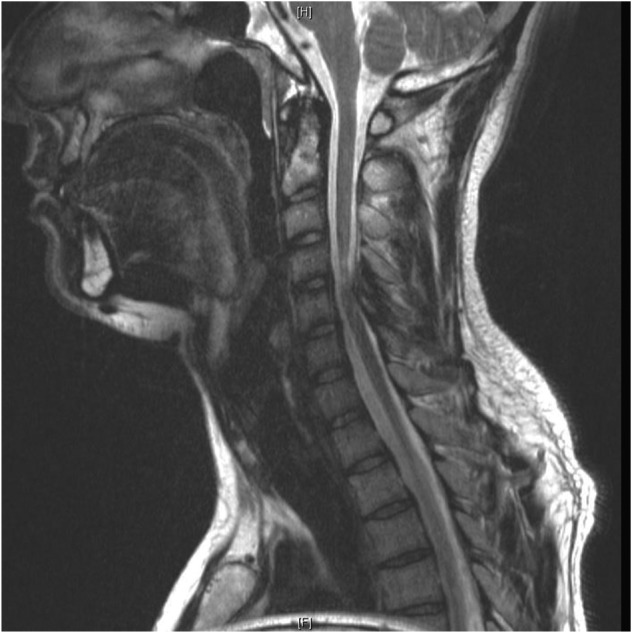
Sagittal T2 weighted MR image of the cervical spine showing a narrowed spinal canal due to a low signal intensity mass anterior to the posterior elements with cord oedema at C4- C6 levels.
